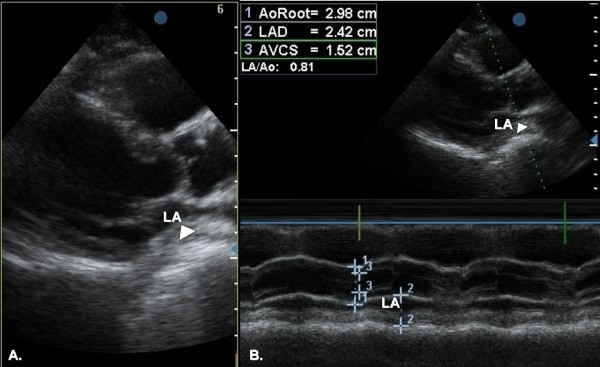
Two dimensional transthoracic echocardiogram. A. Echocardiogram in parasternal long-axis shows an echolucent, apparent left atrial (LA) mass (arrowhead). B. M-mode scan through the ascending aorta and left atrium demonstrates the left atrial cavity significantly confined by the apparent mass (arrowhead).
